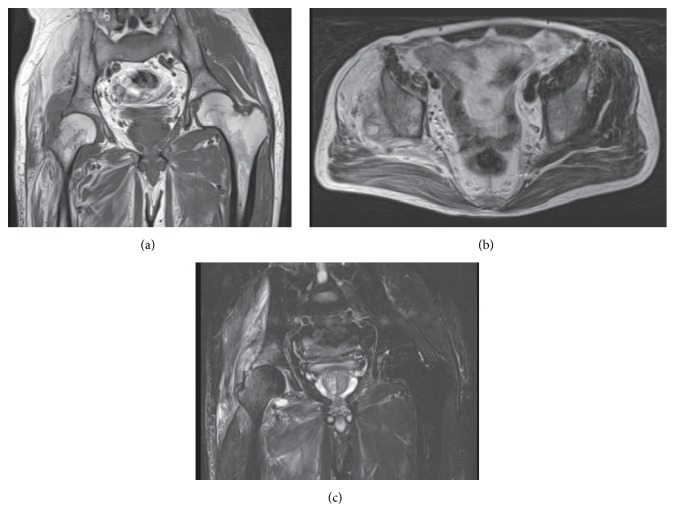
Initial MRI of the hip: (a and b) coronal T1-weighted and axial T2-weighted images showing inhomogeneous fatty signal changes in the gluteus medius muscle and (c) coronal STIR image showing apparent high signal lesions in both the gluteus medius and minimus muscles and slightly high signal lesions in both the femoral head and the acetabular bone with the joint effusion.
